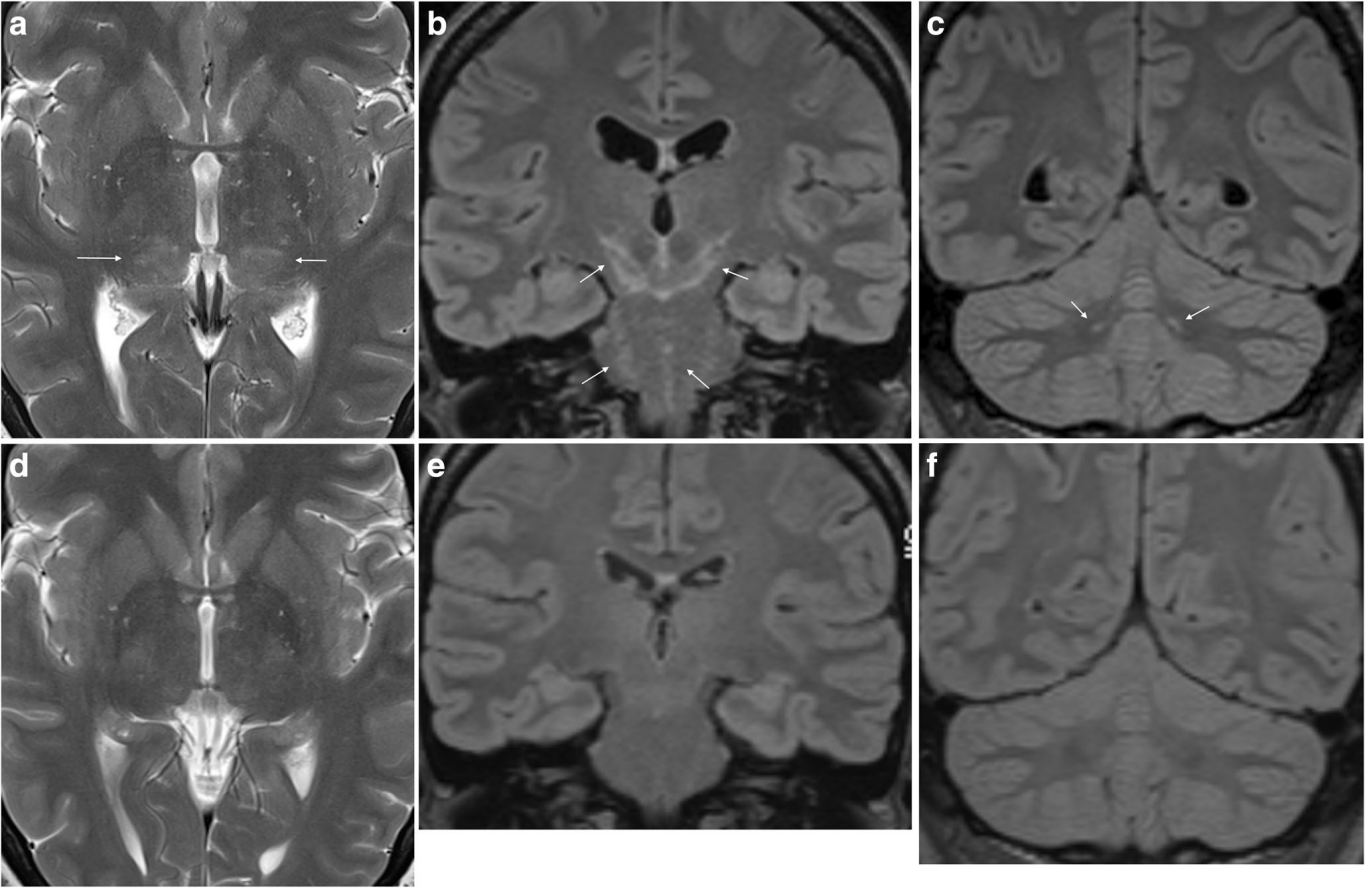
LNB encephalitis in a 40-year-old woman. She presented with 1 day history of headache, vomiting, dizziness and personality change. The CSF cell count was 182 cells/mm^3^. MRI showed symmetric diffuse and patchy T2 (a) and FLAIR (b, c) hyperintensity of inferior thalami (arrows in a), cerebral peduncles, mesencephalon, pons (arrows in b) and cerebellar dentate nuclei (arrows in c). There were no abnormalities on diffusion-weighted or post-contrast images (not shown). Further clinical and laboratory investigations revealed erythema migrans on the right thigh and a positive CSF *Bb* IgG AI. Tests for TBE antibodies were negative. She received antimicrobial treatment, and repeated MRI 8 weeks after the initial scan was normal (d–f)
